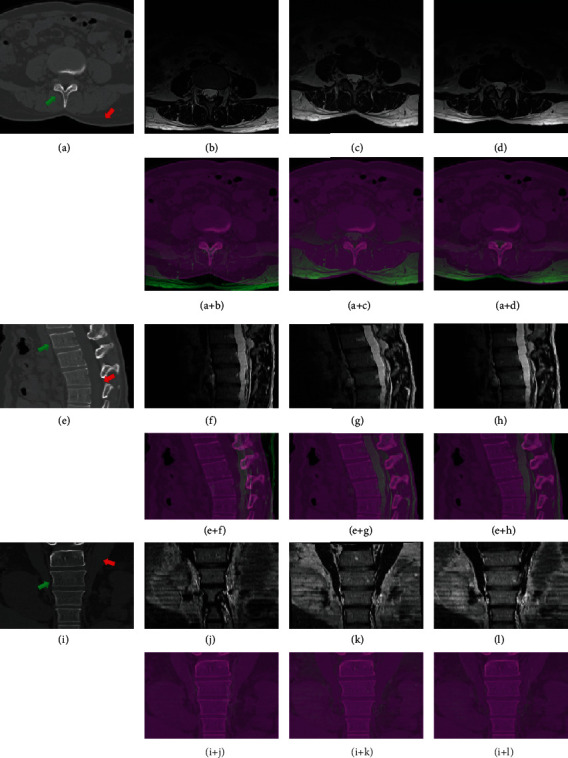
Perceived visual difference of CT-MR images before and after image registration. The regions directed by the arrows are for comparison before and after registration. In addition, images are cropped for display purpose.
